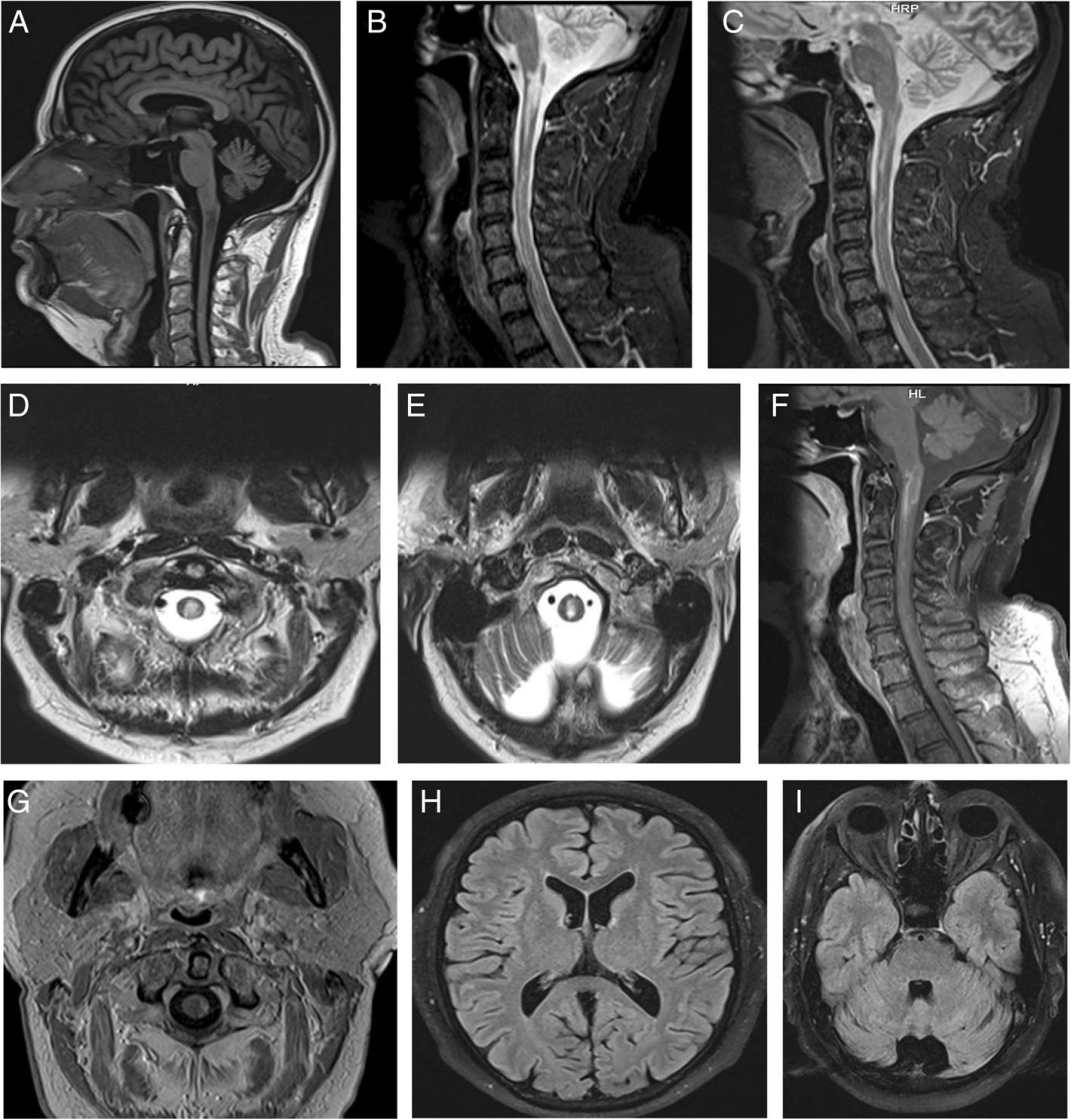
Spinal and cerebral MRI: a sagittal T1 image, b and c sagittal T2 images, d and e axial T2 images show spinal T1 hypointense and T2 hyperintense lesion extending over the first three cervical vertebrae and abutting medullary area postrema. f and g sagittal and axial T1 images with contrast show marginal enhancement. h and i axial flair images show normal cerebrum and cerebellum
